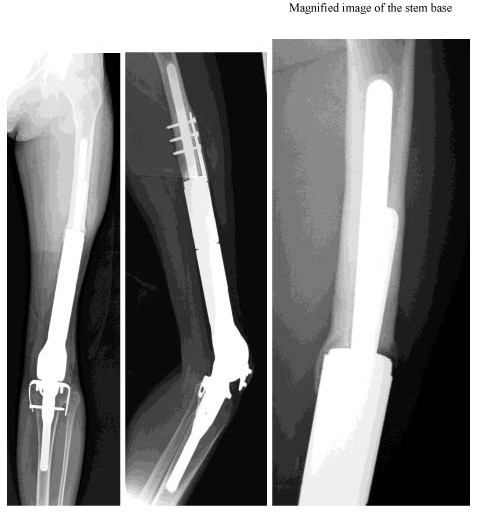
Plain X-ray films at the time of stem fracture.
